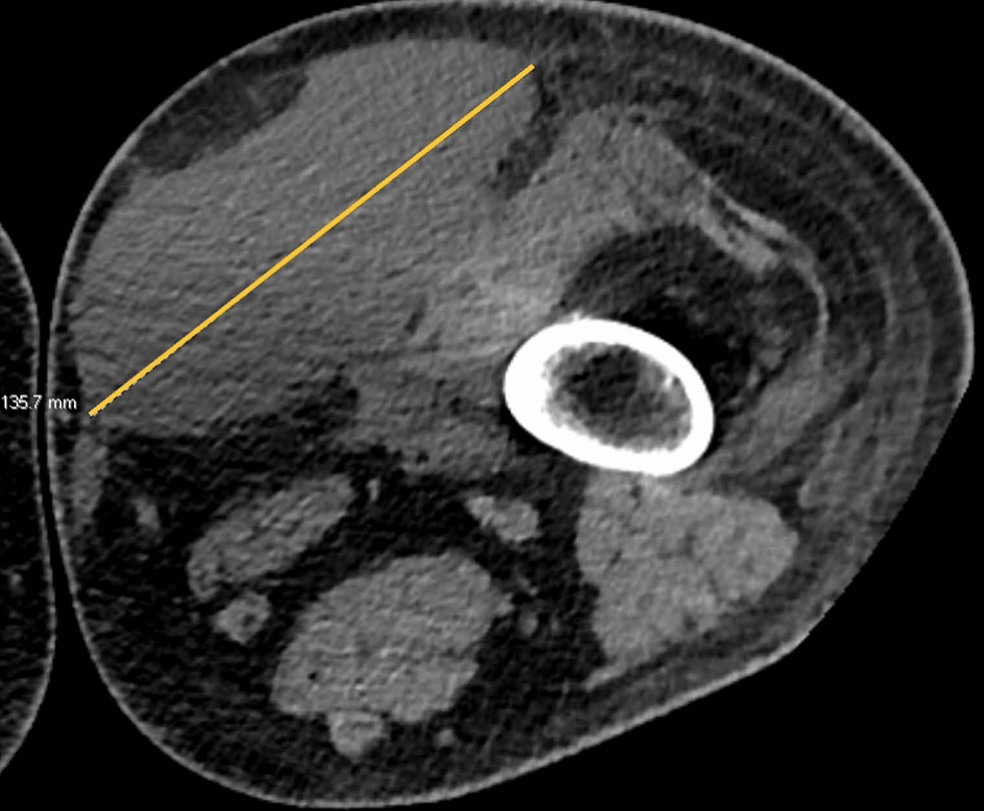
October 2022 CT left lower extremity with contrast shows large anteromedial right thigh deep subcutaneous fluid collection, 43.4 x 13.6 x 5.4 cm in craniocaudal/transverse/anteroposterior dimensions, likely representing the Morel Lavallee lesion. This image shows the transverse dimension.
